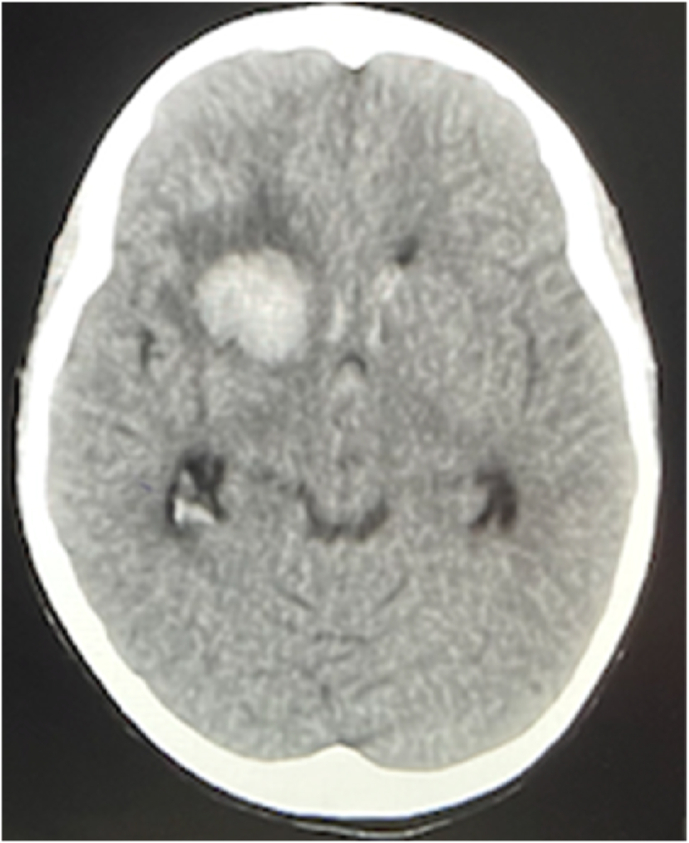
Non-contrast CT study shows right sided basal ganglia hyperdense hemorrhage noted with mass effect ipsilateral lateral ventricle and related perifocal edema. Extension of the hemorrhage into the ventricular system.
